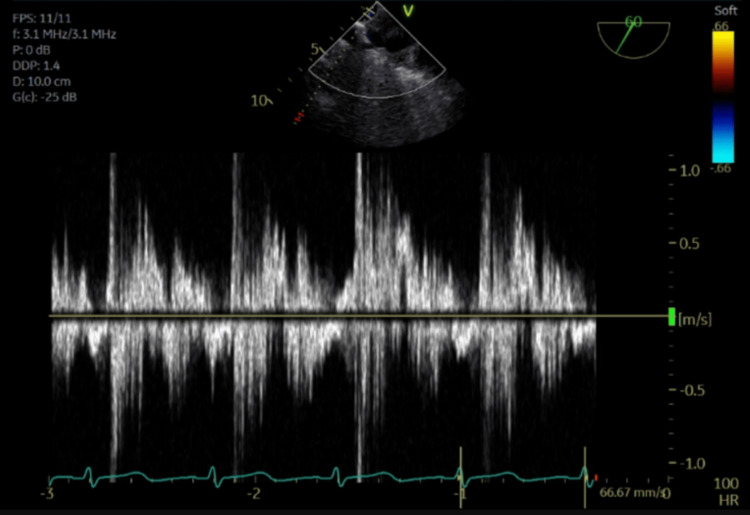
Pulsed-wave Doppler of the right inferior pulmonary vein showing systolic flow reversal.
